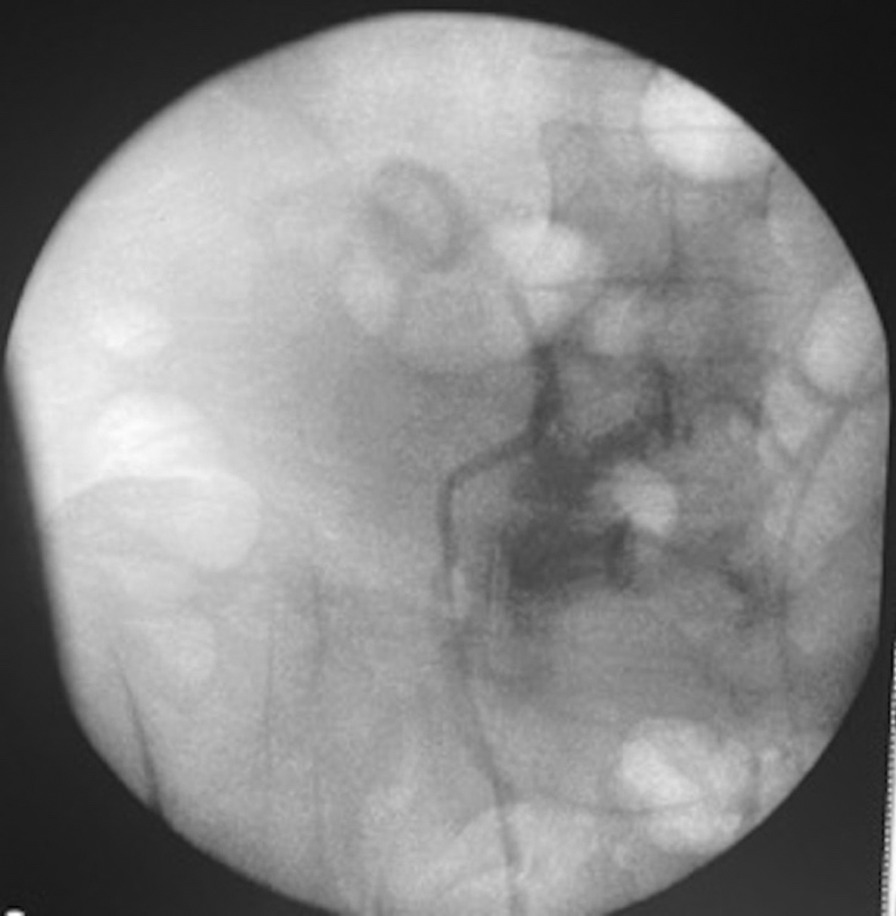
Fluoroscopic retrograde urography showing right renal pelvis dilation with a filling defect consistent with the calculus noted on sonogram
